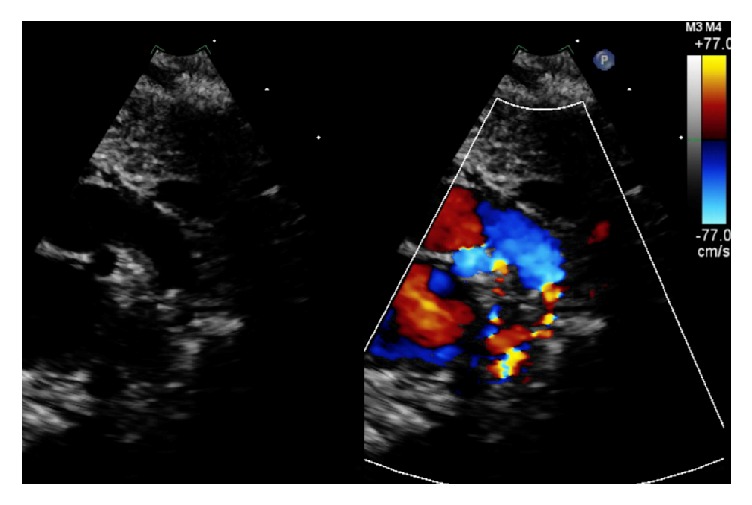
Echocardiogram demonstrating juxtaductal coarctation of the aorta following patent ductus arteriosus ligation surgery.
